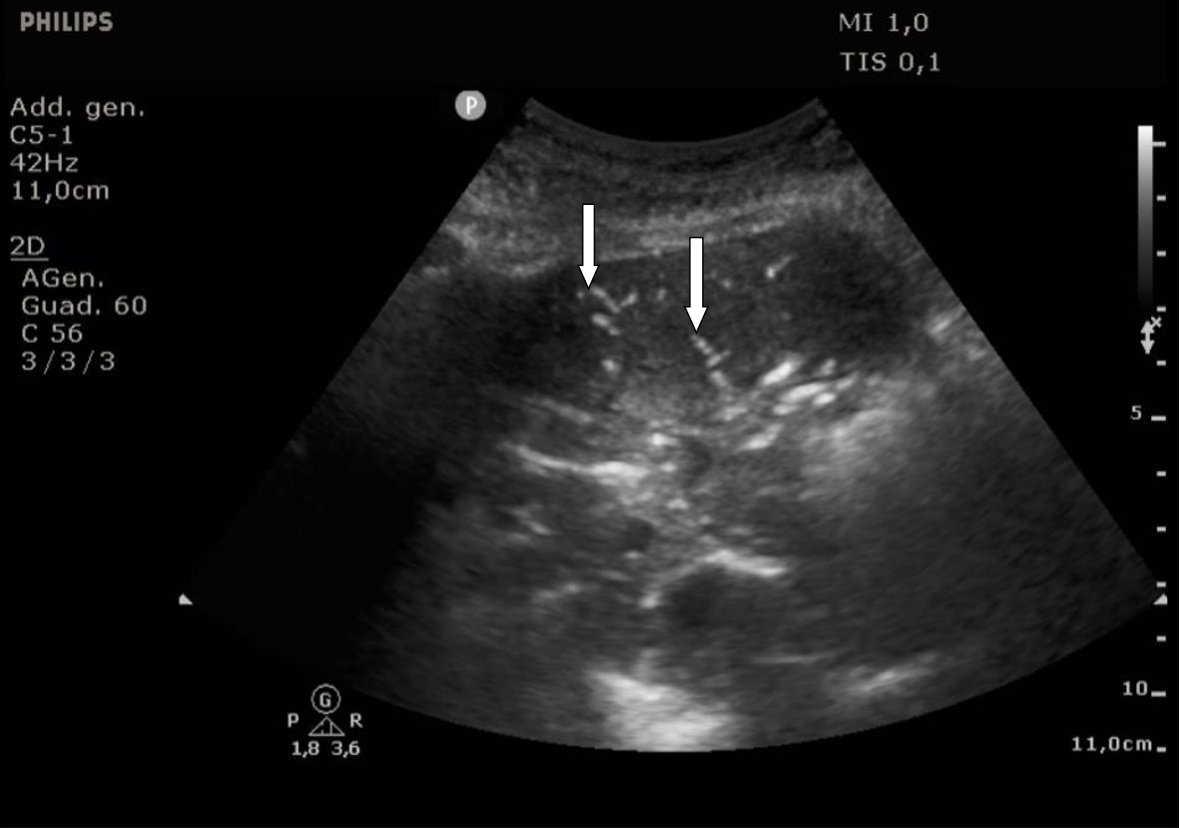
Sonographic air broncograms. The longitudinal US scan of the thorax, obtained at the level of the mid-axillary line with a convex probe, shows a large post-operative pneumonia. Two air-bronchograms are visible as two linear hyperechoic findings (white arrows)
